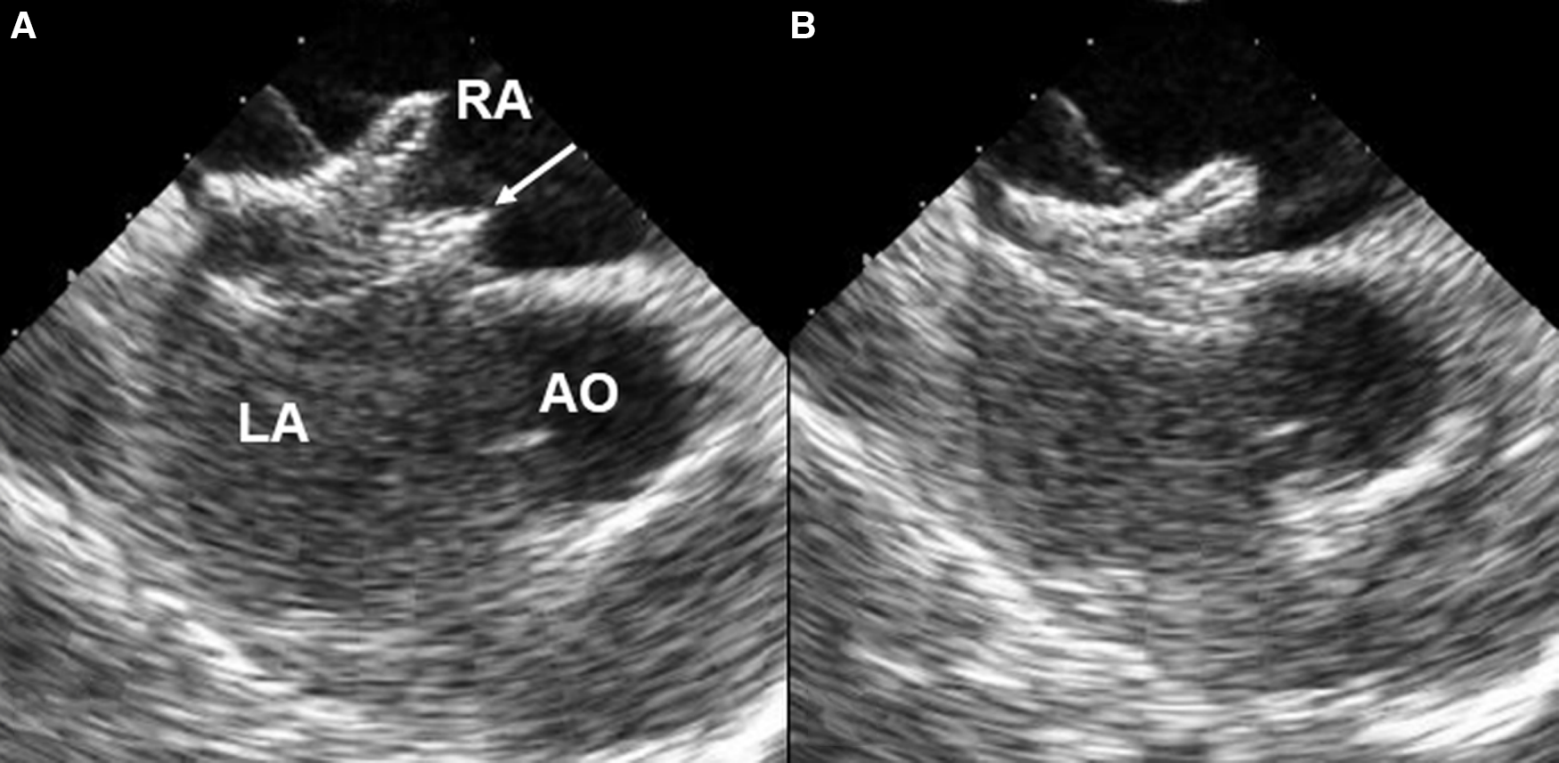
Intracardiac echocardiography showing the prolapsed superioanterior part (arrow) of left atrial disc (A) which was successfully reduced (B) with the push back technique. AO, aorta; RA, right atrium; LA, left atrium.
